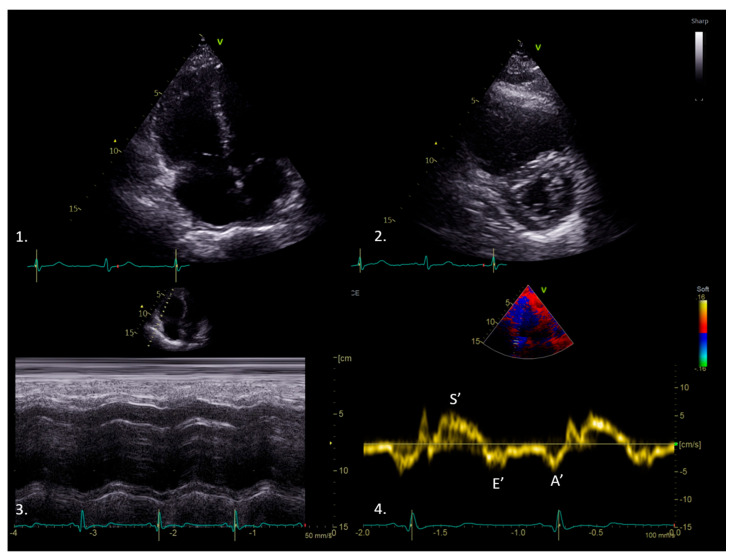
Transthoracic echocardiography of a patient with massive pulmonary embolism showing right ventricular dilatation (1); signs of pressure overload (2); reduced longitudinal function, as demonstrated by a reduced tricuspid annular plane systolic excursion (3); and reduced systolic velocity (S’) of the right ventricular lateral wall in tissue Doppler imaging (4).
